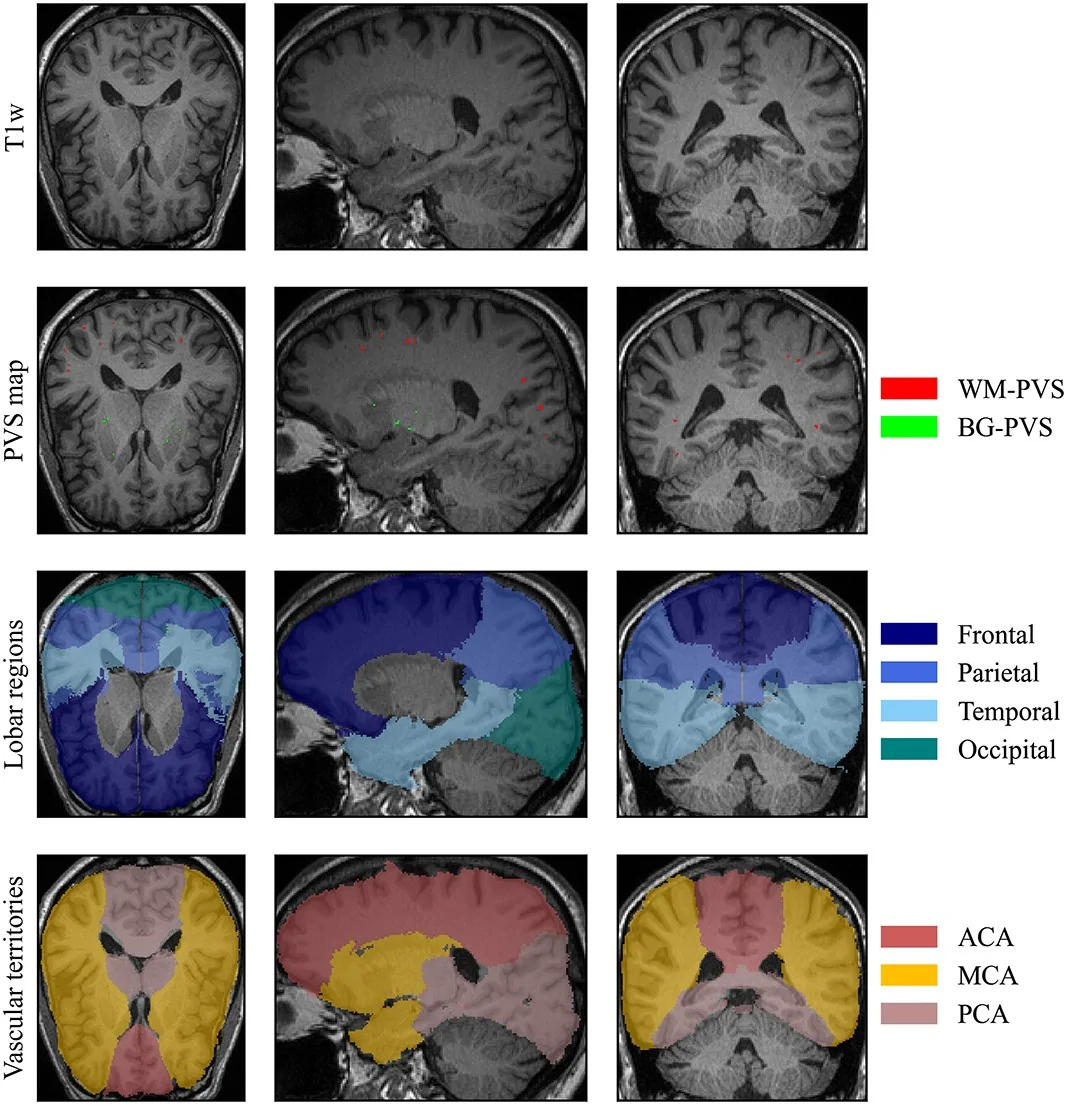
Examples of T1w MRI with segmented PVS maps and region atlas parcellations according to lobar regions and arterial vascular territories. Top row: Raw T1w MRI scan from a stroke-free participant with images from the axial, sagittal, and coronal planes. Second row: PVS map overlaid on T1w MRI, with WM-PVS and BG-PVS. Third row: Lobar regions including the frontal, parietal, temporal and occipital lobes. Bottom row: Arterial vascular territories including the regions supplied by the ACA, MCA and PCA. Abbreviations: ACA = anterior cerebral artery; BG = basal ganglia; PCA = posterior cerebral artery; PVS = perivascular spaces; T1w = T1-weighted; WM = white matter.
